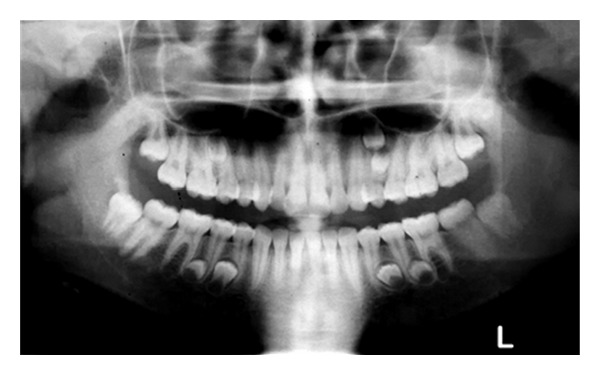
Panoramic radiograph of the patient showing the presence of seven supernumerary premolars.
